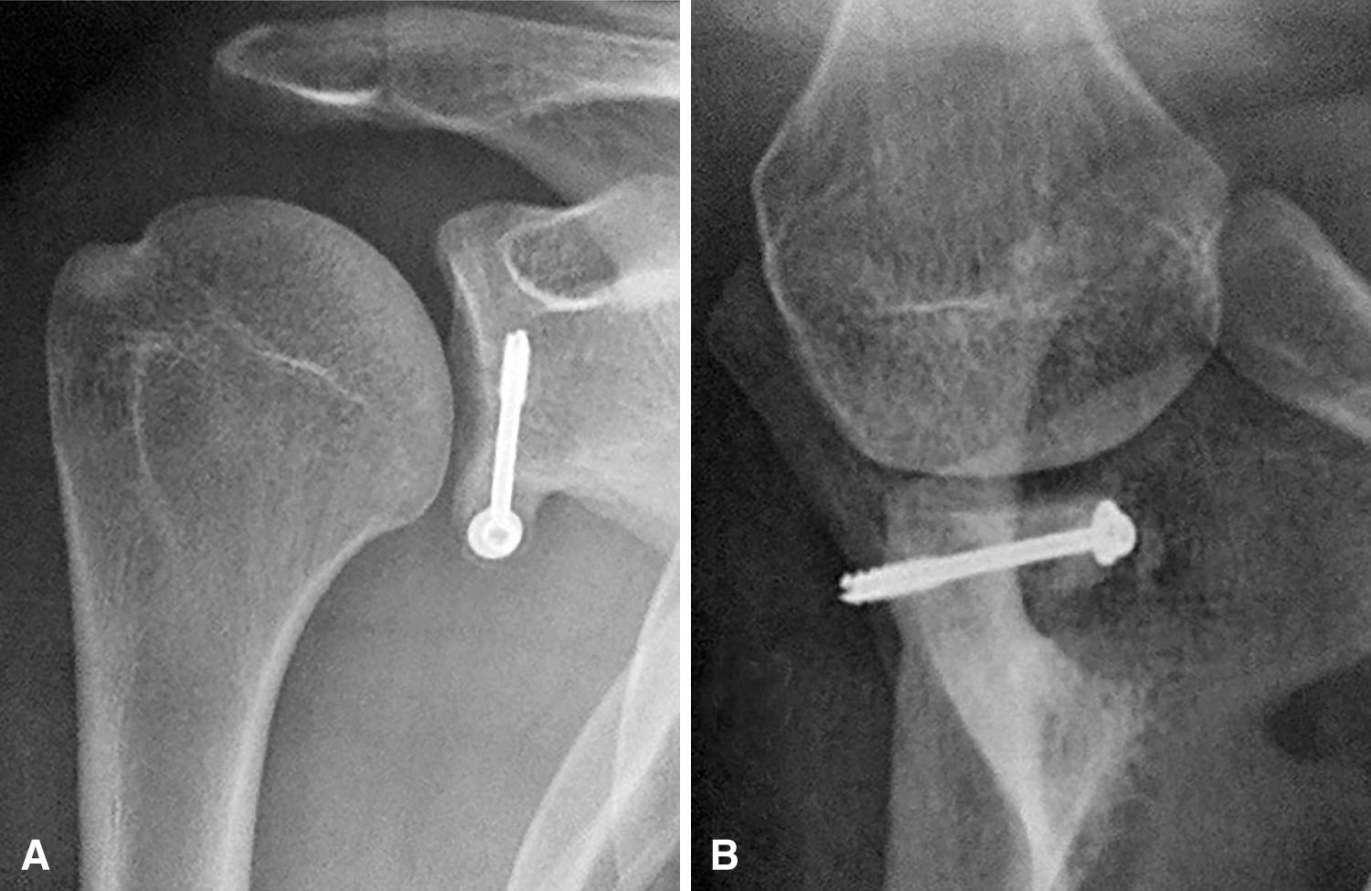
An example of coracoid graft optimal position and healing is shown. Postoperative (A) AP and (B) lateral radiographs demonstrate optimal positioning of the coracoid bone graft: below the equator and flush to the glenoid surface, restoring the concavity of the glenoid with perfect bone healing.
